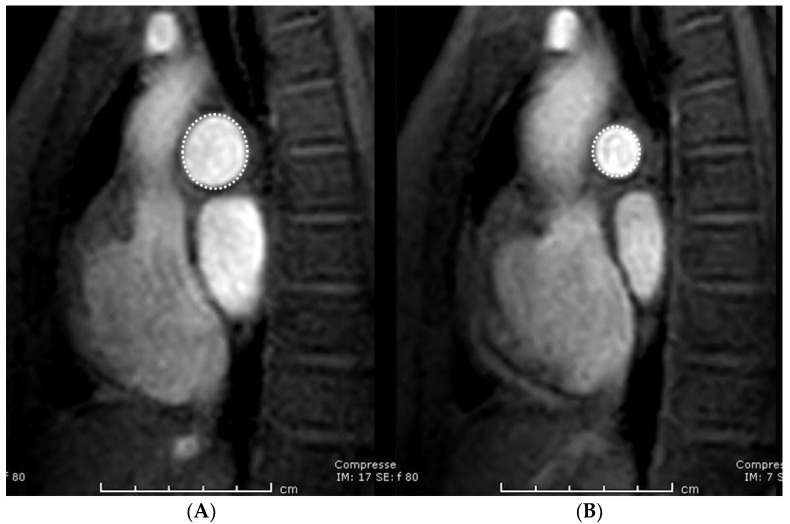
Phase-contrast magnetic resonance imaging of the pulmonary artery demonstrates the measurement of maximum area (A) and minimum area (B).
